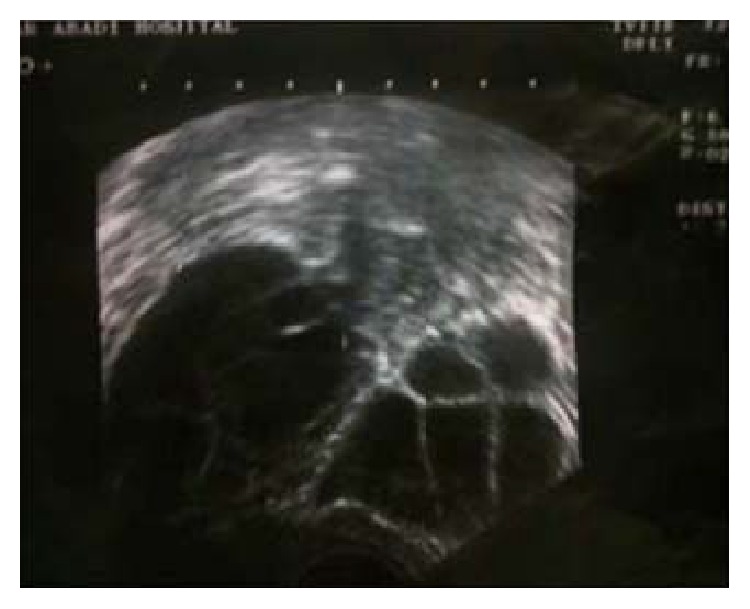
Ultrasound image shows bilateral ovarian multilocular cysts extending to the midabdomen.
